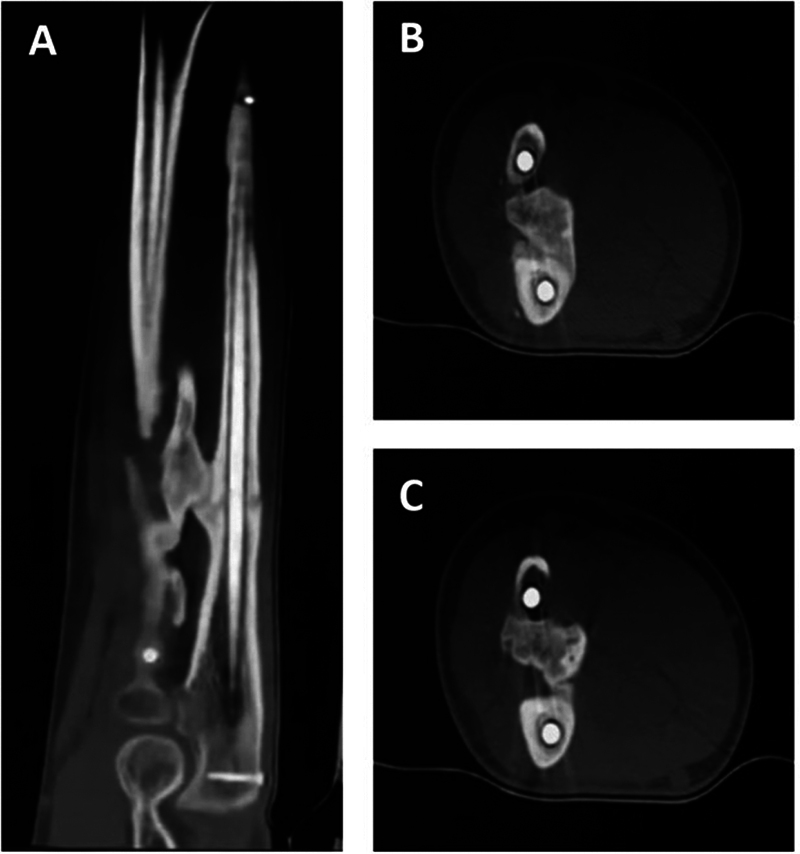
CT scan images displaying the radioulnar synostosis at the junction of the middle third with the proximal third of the diaphysis. Sagittal slices (A), axial slices (B, C).
